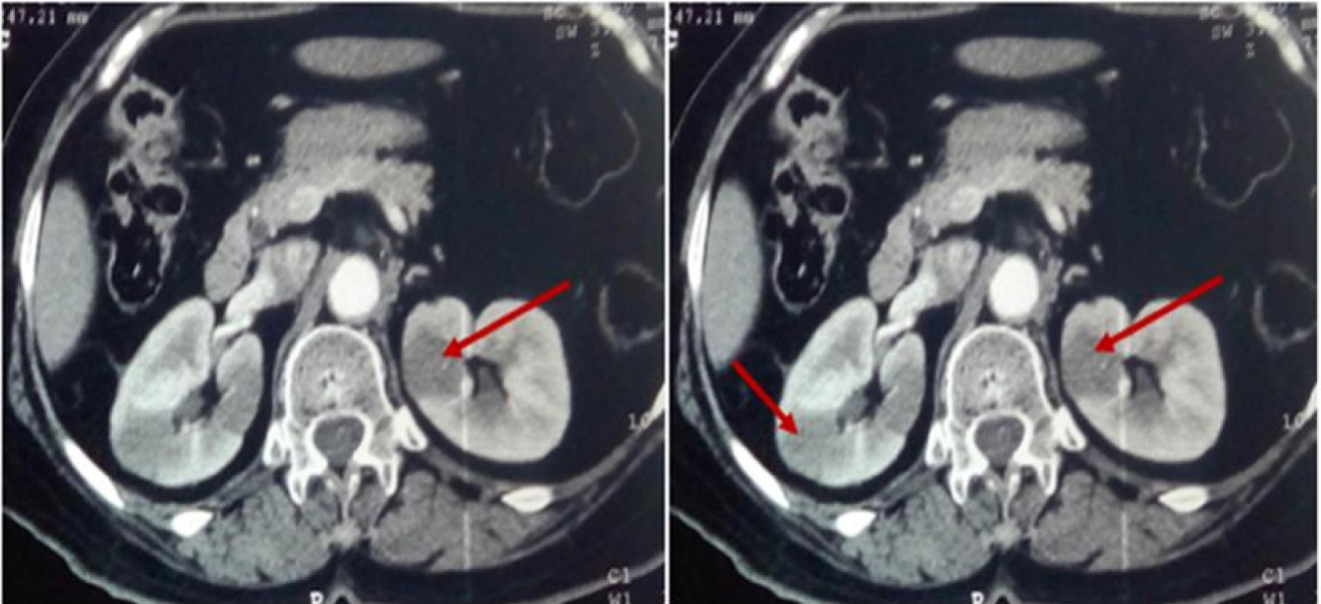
CT scan showing bilateral renal lesions.
